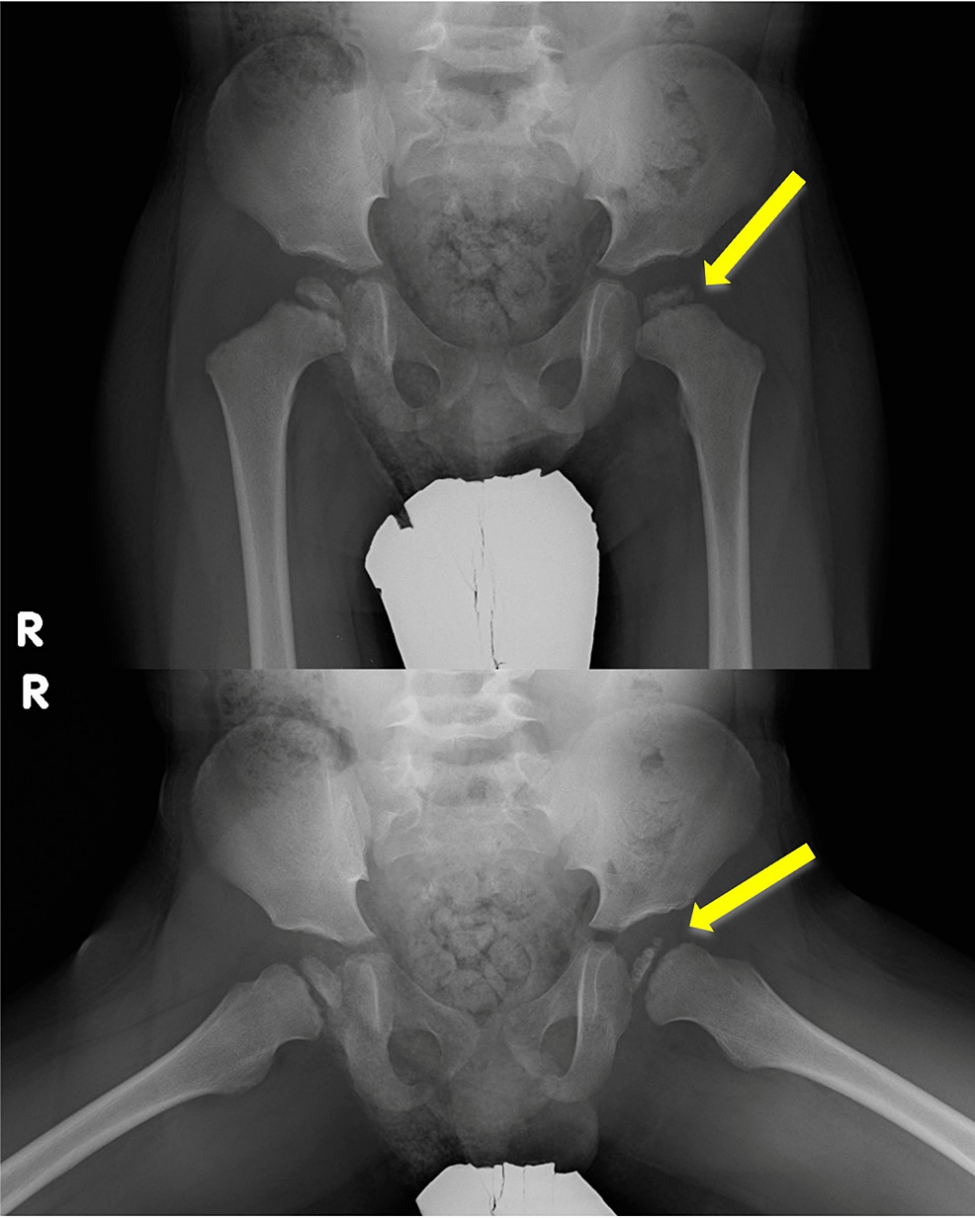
Pelvic X-ray scan showing thinning of the left femoral head (yellow arrows).
